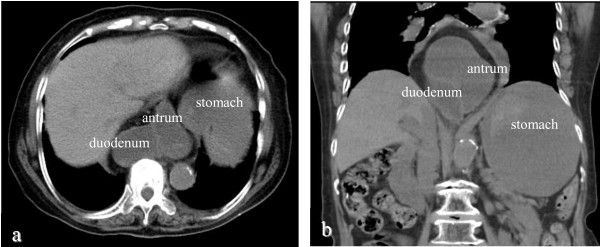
Plain abdominal computed tomography. The prolapse of the gastric antrum and duodenal bulb into the mediastinum were revealed. The stomach and duodenal bulb were markedly dilated and retention of fluid contents was evident (a: axial section, b: coronal section).
